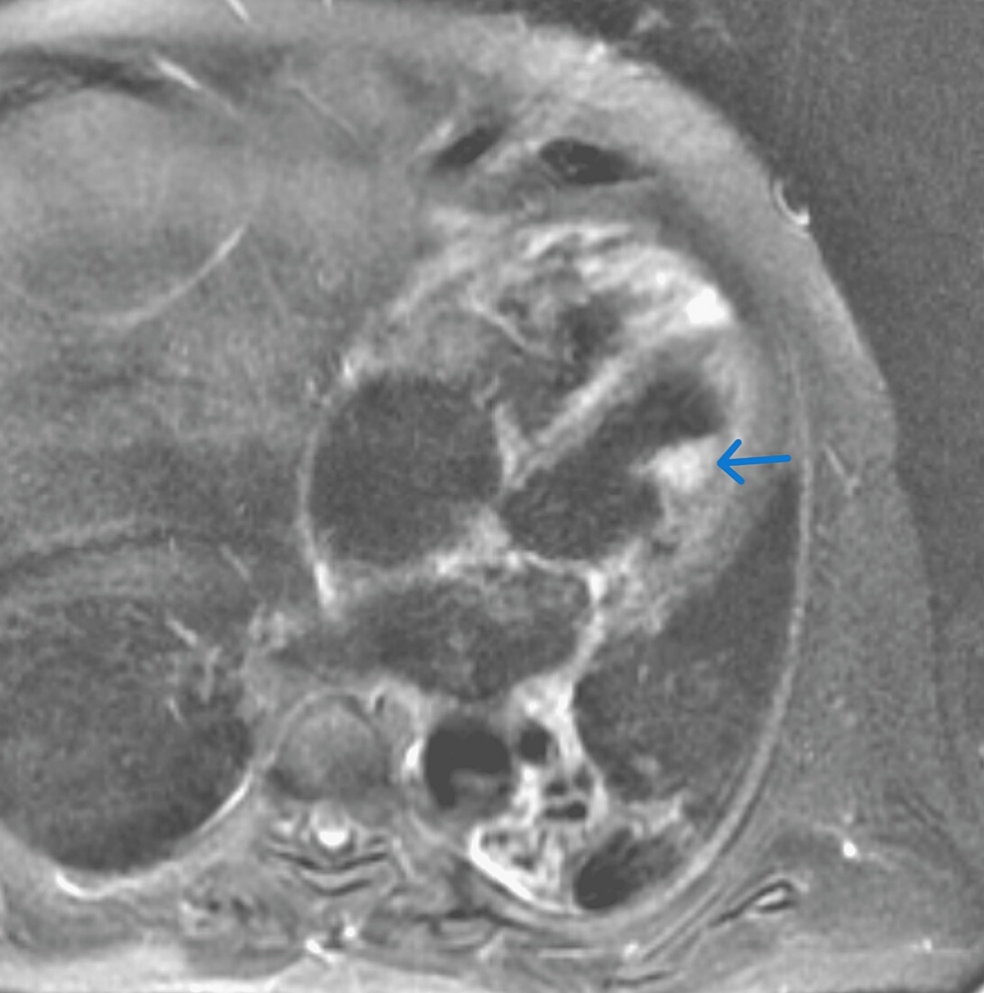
Cardiac MRI showing left ventricular hyperintense mass compared to surrounding myocardium.
